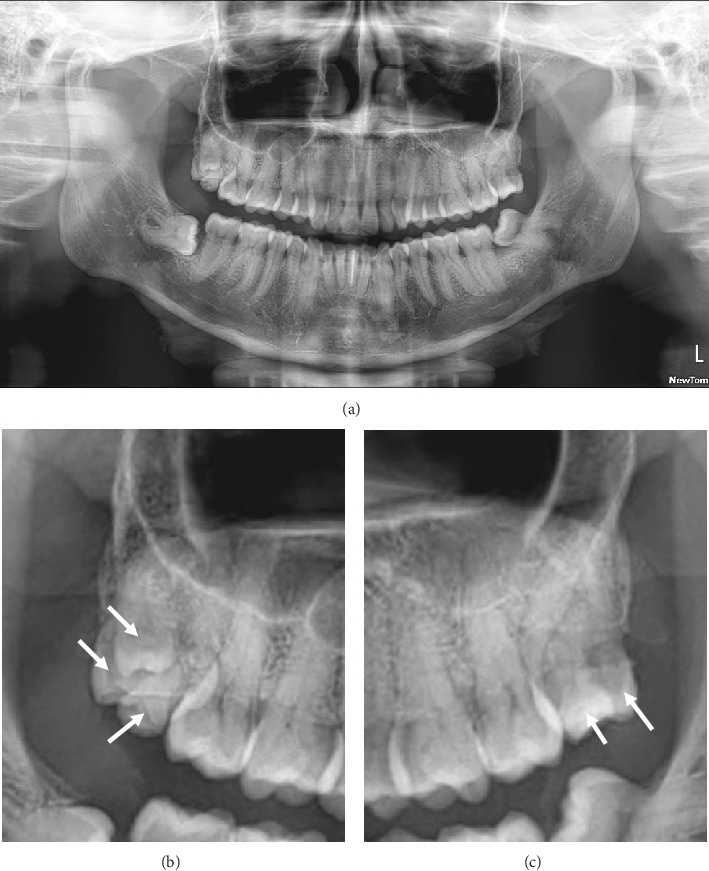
(a) Panoramic radiograph of a 38-year-old male patient showing the presence of maxillary supernumerary molars in each hemiarch. (b) Screenshotted panoramic radiograph of the right maxillary quadrant. (c) Screenshotted panoramic radiograph of the left maxillary quadrant. The white arrows point to the left third molar and the supernumerary fourth molar.
